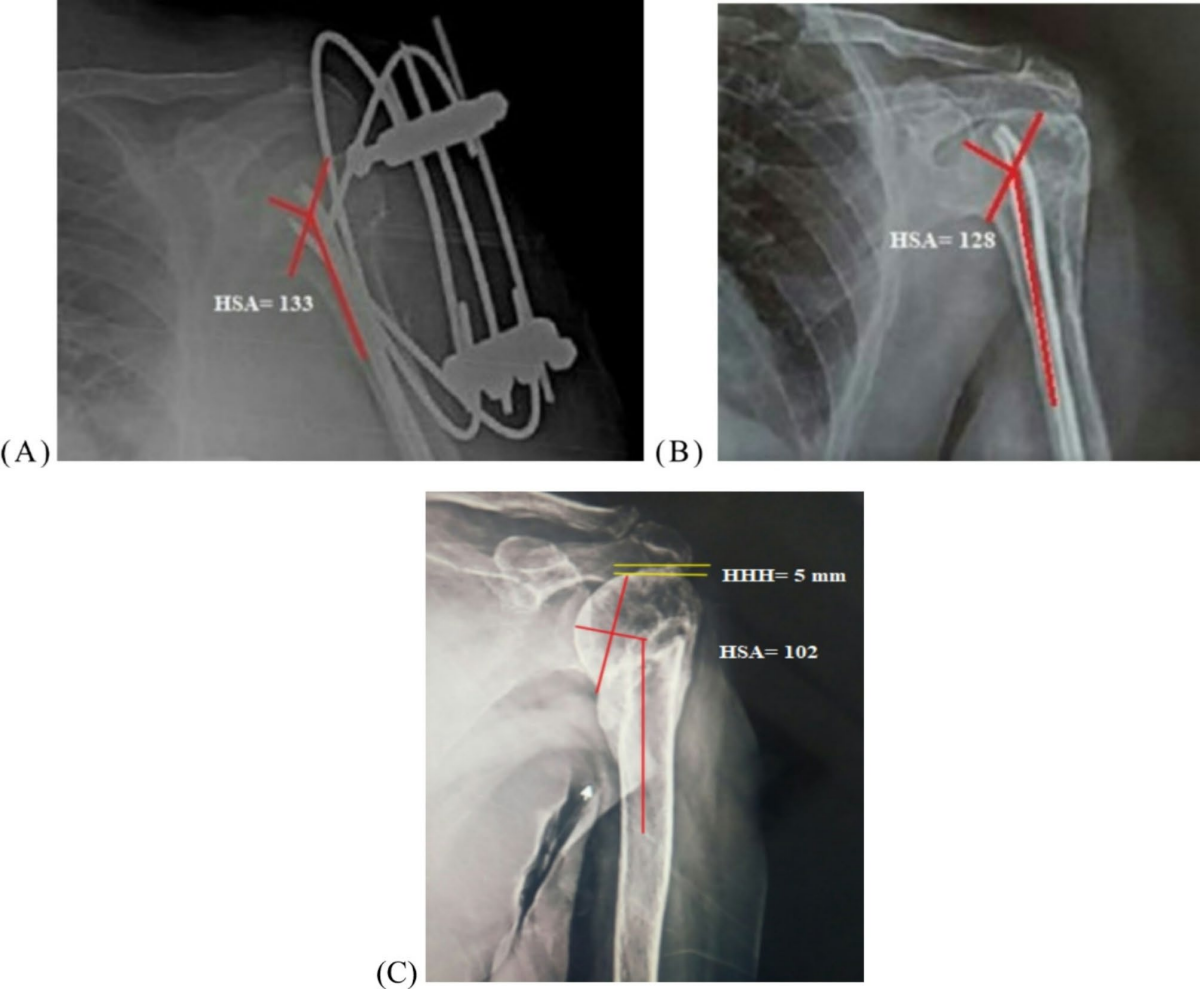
The HSA angle in the initial radiograph is 133° (A) and 128° at 12 months postoperative (B) after modified MIROS fixation. The HHH was − 5 mm, and the HSA was 102° at one-year radiograph following conservative treatment, indicating varus malunion (C)
